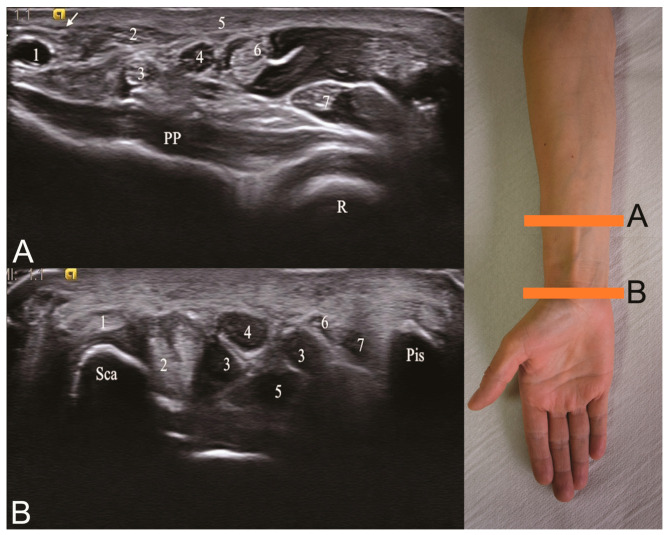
The median nerve ultrasound in the transverse plane at two different sites. The cross-sectional area (CSA) of the median nerve was measured at the wrist and 5 cm proximal to this level. (A) R—radius bone, PP—pronator quadratus muscle, (1) radial artery, (2) flexor carpi radialis tendon, (3) flexor pollicis longus tendon, (4) median nerve, (5) palmaris longus tendon, (6) flexor digitorum superficialis tendon, (7) flexor digitorum profundus tendon, arrow—flexor retinaculum. (B) Sca—scaphoid bone, (1) flexor carpi radialis tendon, (2) flexor pollicis longus tendon, (3) flexor digitorum superficialis tendon, (4) median nerve, (5) flexor digitorum profundus tendon, (6) ulnar artery, (7) ulnar nerve, Pis—Pisiform bone.
